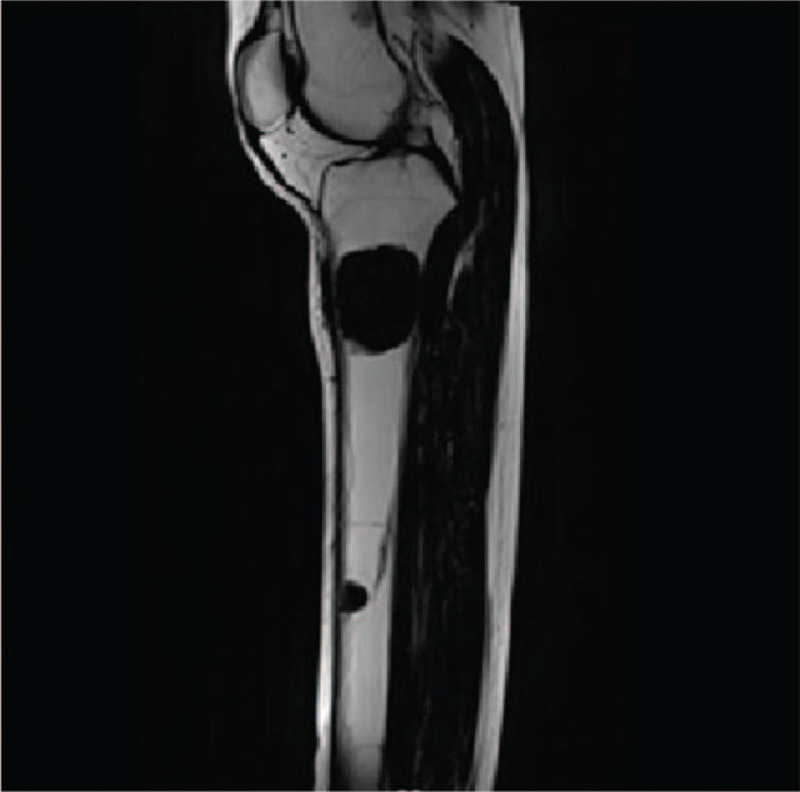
MRI of the patient showed a soft/hard tissue mass (37 mm × 30 mm × 42 mm) in the anterior/posterior compartment of the left lower leg.
